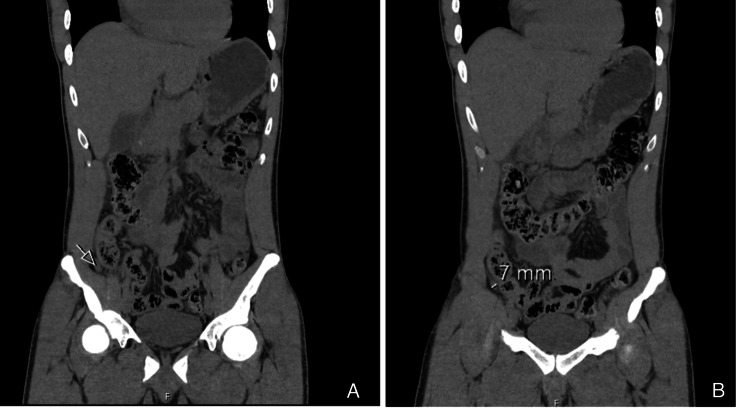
Coronal CT scan showing inflammatory changes in the right iliac fossa (A) and a 7 mm appendix (B)
